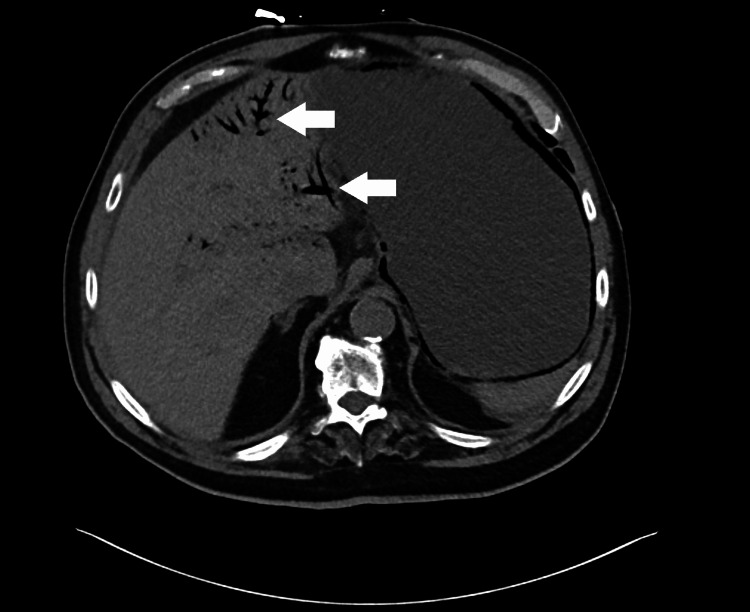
CT abdomen demonstrating portal venous gas Hypodense air present within the portal venous system is highlighted by the arrows.
